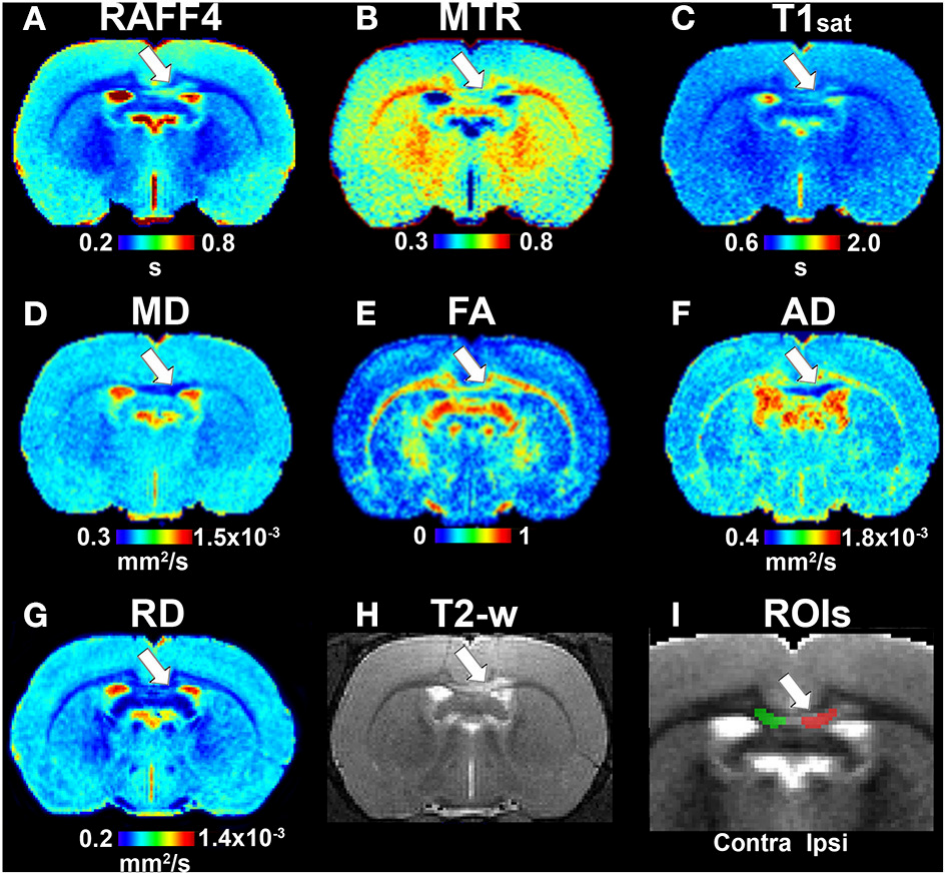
Quantitative MRI maps in the demyelination phase, on day 3: RAFF4 (A), magnetization transfer ratio, MTR (B), T1sat (C), mean diffusivity, MD (D), fractional anisotropy, FA (E), axial diffusivity, AD (F), radial diffusivity, RD (G), T2w image with lesion (H) and representative example of ROIs for analyzing the lesion on a grayscale RAFF4 map (I). White arrow points to the lesion in the corpus callosum.
